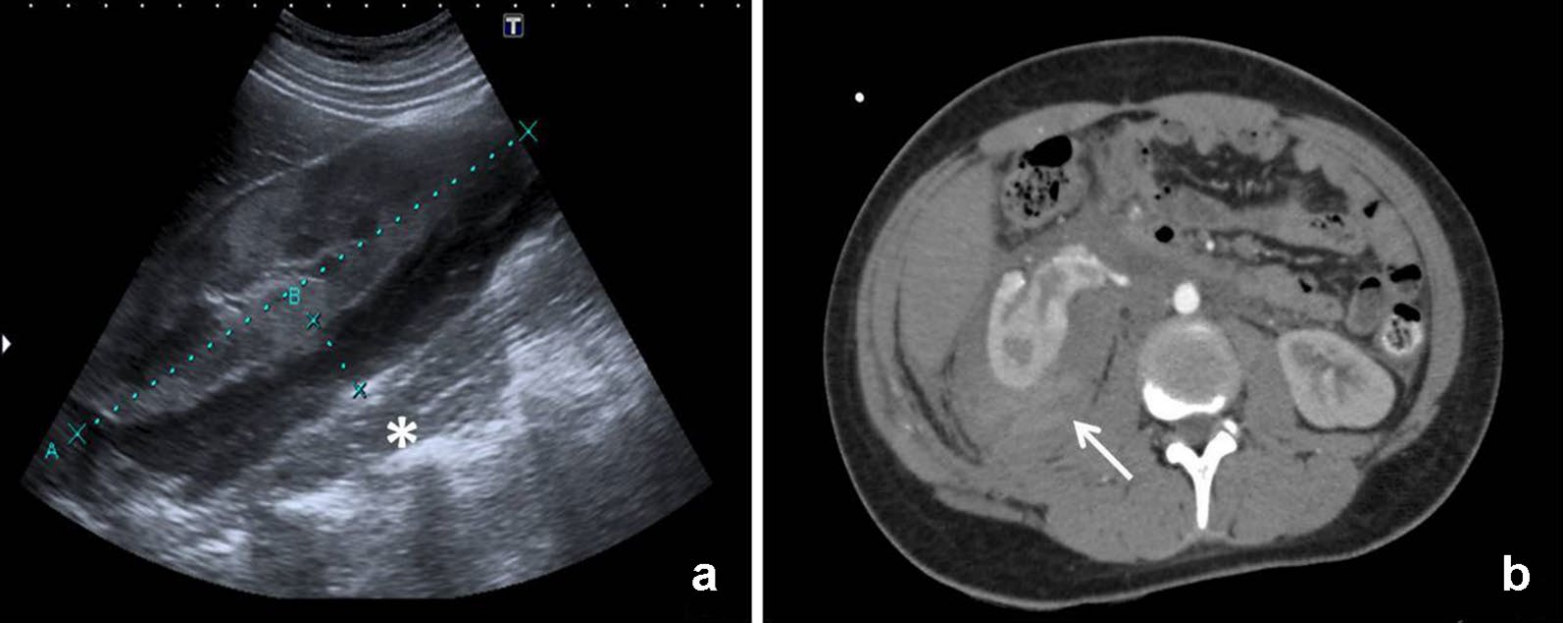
a r-EFAST: perirenal fluid collection anterior to psoas muscle (*asterisk*) measuring approximately 14 × 2 cms (callipers). b CT Scan with intravenous contrast: right perirenal hematoma with different densities that may correspond to active bleeding extending to pararenal space (*arrow*)
